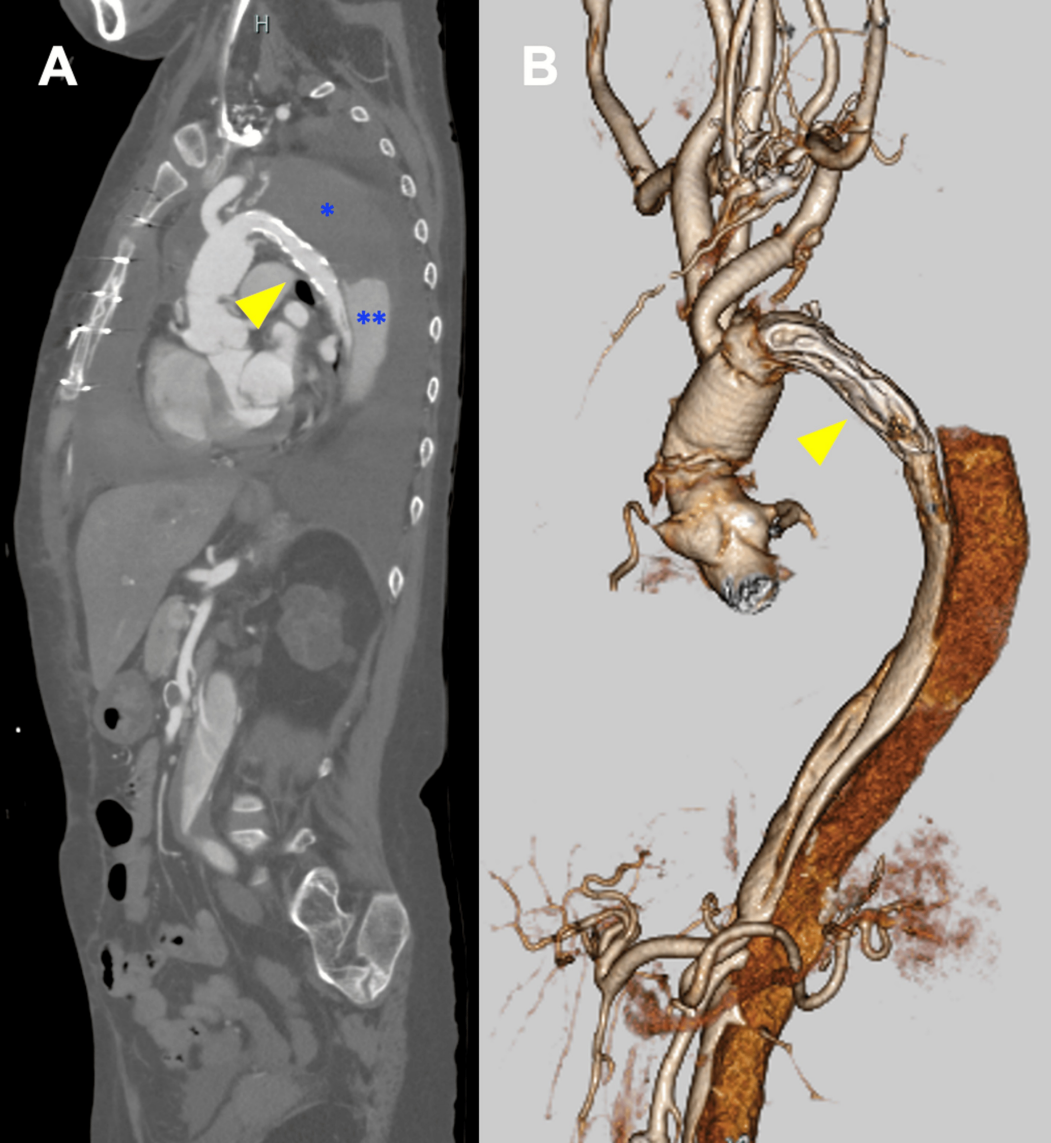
CT images after the initial surgery A. Sagittal image. B. 3D CT image. Thrombosis of the former false lumen is observed in the proximal descending aorta (blue asterisk), while contrast enhancement from distal re-entry is seen beyond the mid-descending aorta (double blue asterisk). Despite the insertion of a stent graft as part of the FET, sufficient expansion of the true lumen (yellow arrow) was not achieved CT: computed tomography; FET: frozen elephant trunk
